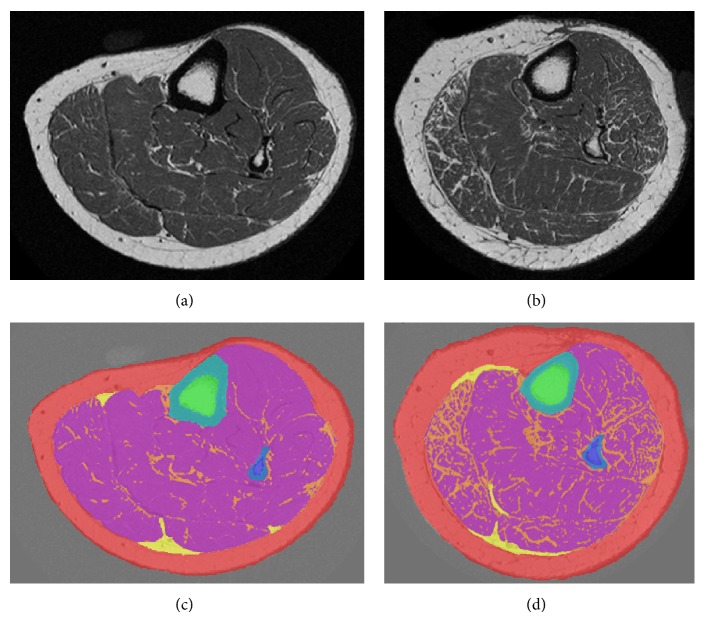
Axial MRI images of the lower leg from a Caucasian postmenopausal woman without type 2 diabetes (age 68 years, percent IntraMAT = 8%) (a) and with type 2 diabetes (age 67 years, percent IntraMAT = 14%) (b). Segmented images of the lower leg from the participant without type 2 diabetes (c) and with type 2 diabetes (d). Subcutaneous adipose tissue (red), muscle (fuchsia), intermuscular adipose tissue (InterMAT) (yellow), intramuscular adipose tissue (IntraMAT) (orange), tibia cortical bone (blue), tibia bone marrow (green), fibula cortical bone (royal blue), and fibula bone marrow (purple).
